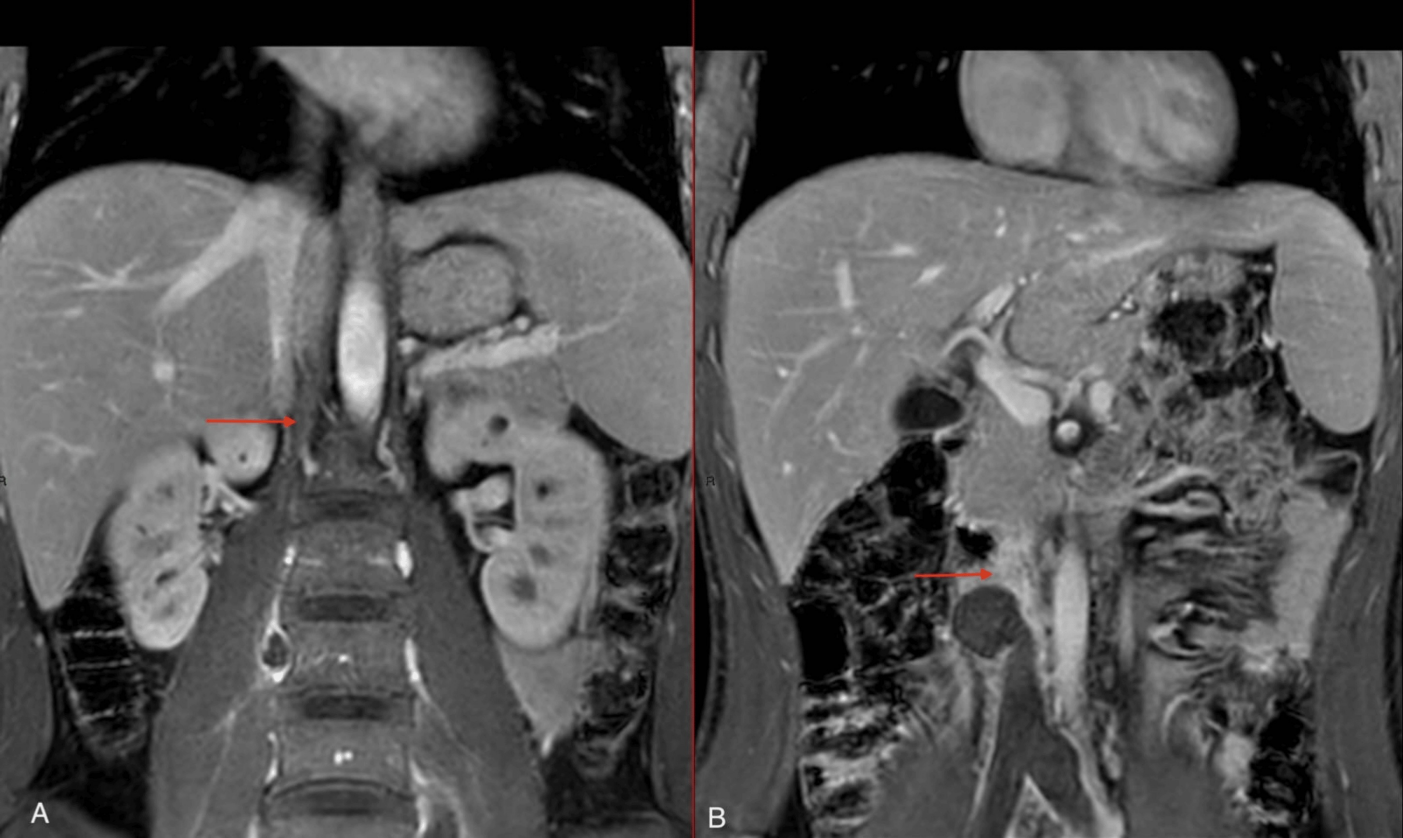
MRI abdomen with contrast - coronal view (A) Atretic infrahepatic and non-opacified IVC and bilateral right and left common iliac veins. (B) Image showing the small caliber of the infrahepatic IVC. IVC: inferior vena cava
